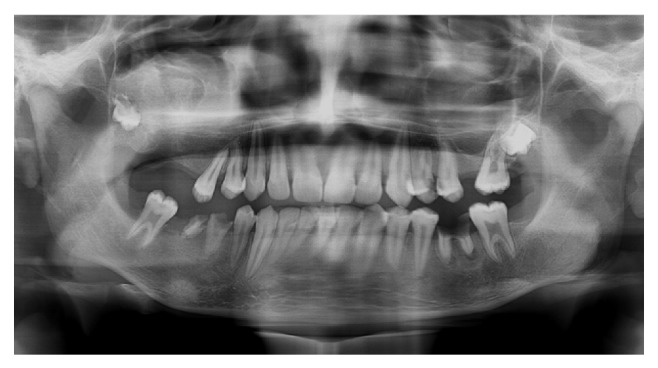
Postoperative radiograph showing signs of calcification in maxilla and condyle.
